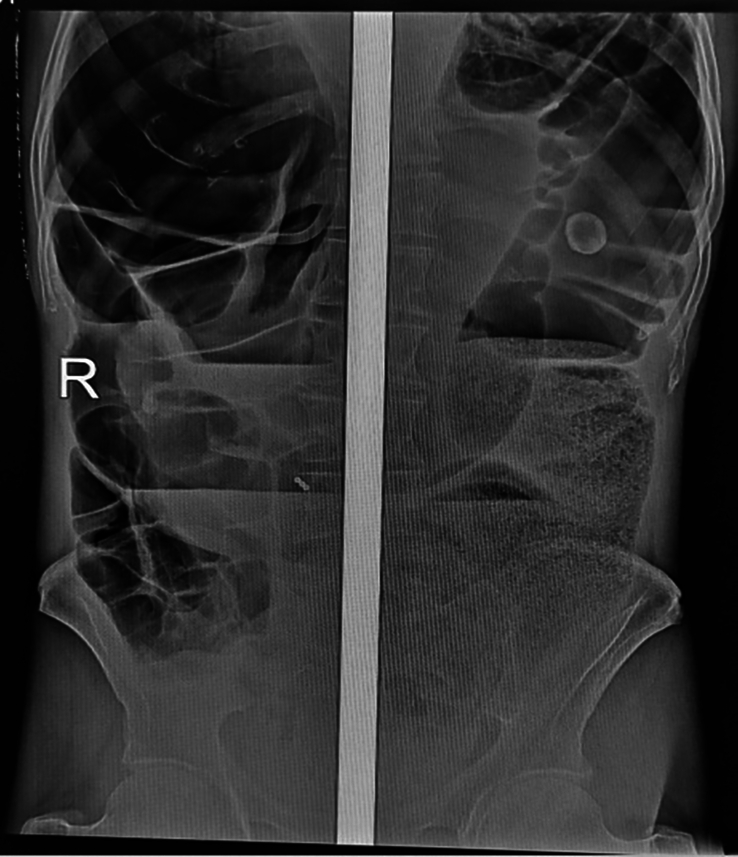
Abdominal X-ray showing a dilated descending colon, transverse colon and caecum loaded with faeces.
